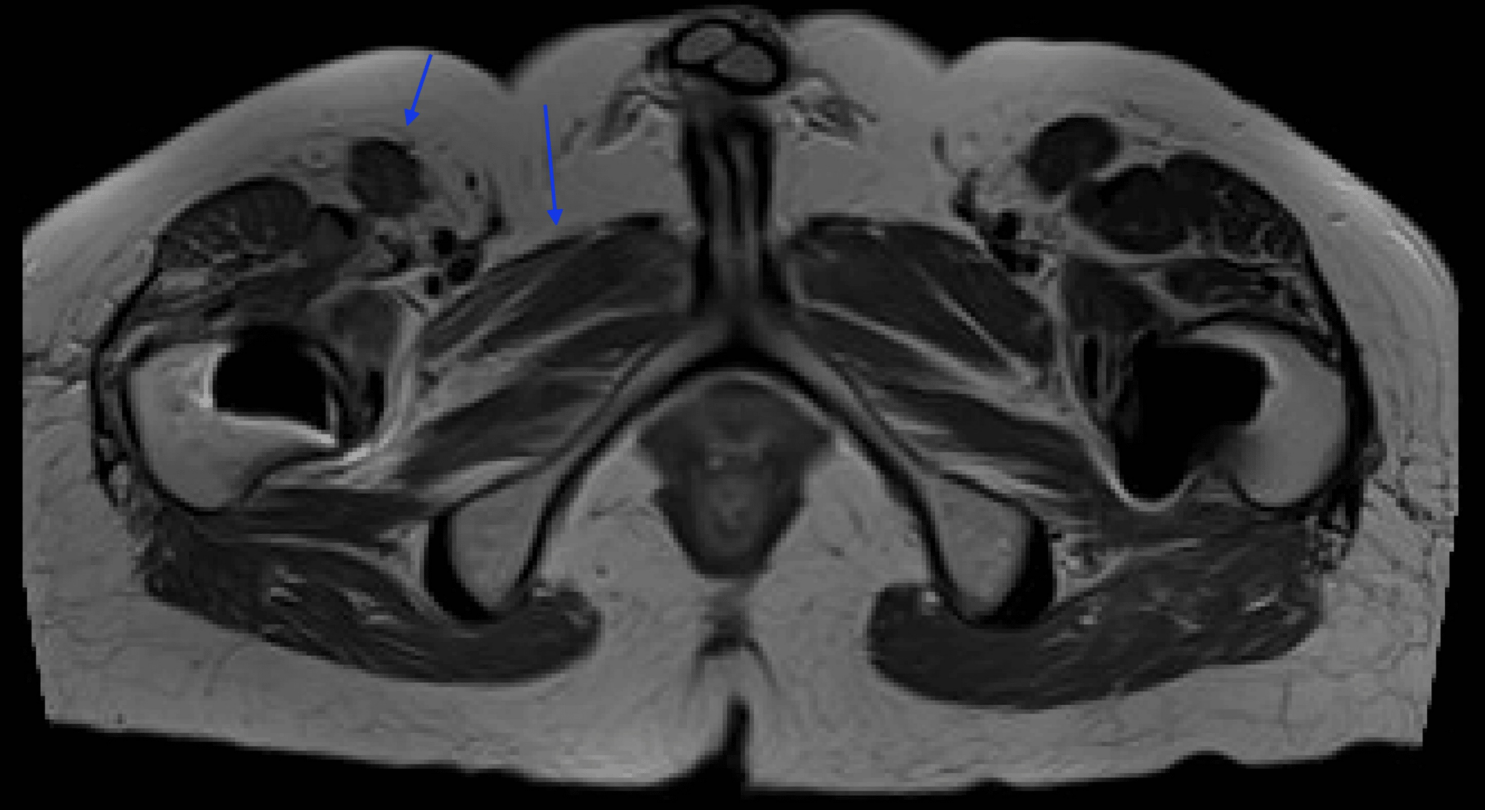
Magnetic resonance imaging showing the atrophied muscles
